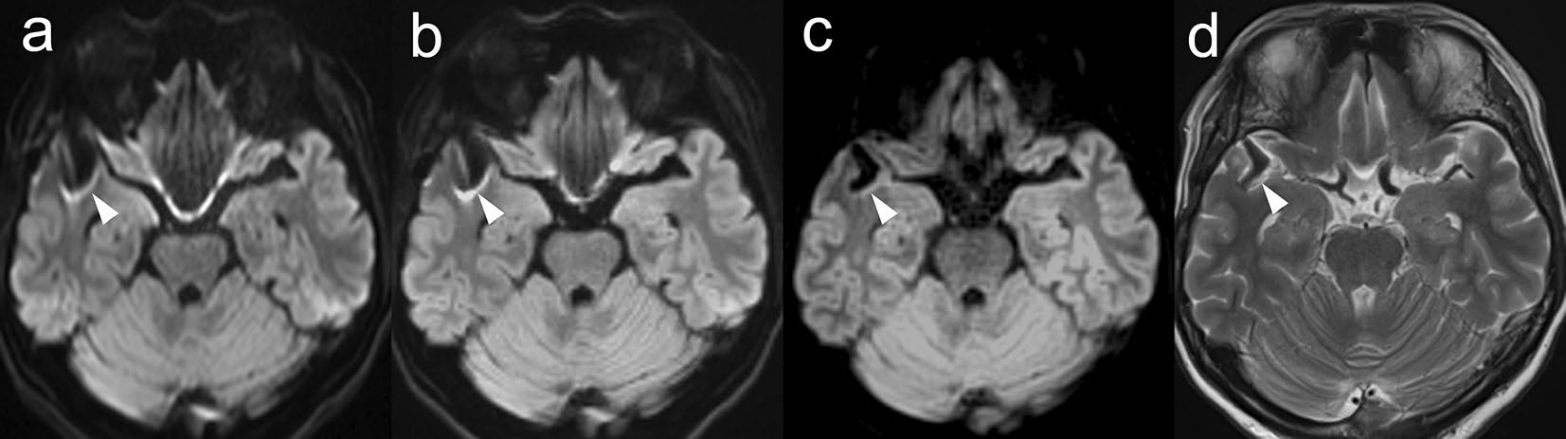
Representative images of SS-EPI DWI (a), RESOLVE DWI (b), TGSE-BLADE DWI (c) and T2WI (d) in a 60-year-old female patient after cerebral aneurysm clipping at the right middle cerebral artery. The arrowheads indicate the clip and the clip-induced artifacts. TGSE-BLADE DWI has the least artifact and distortion.
